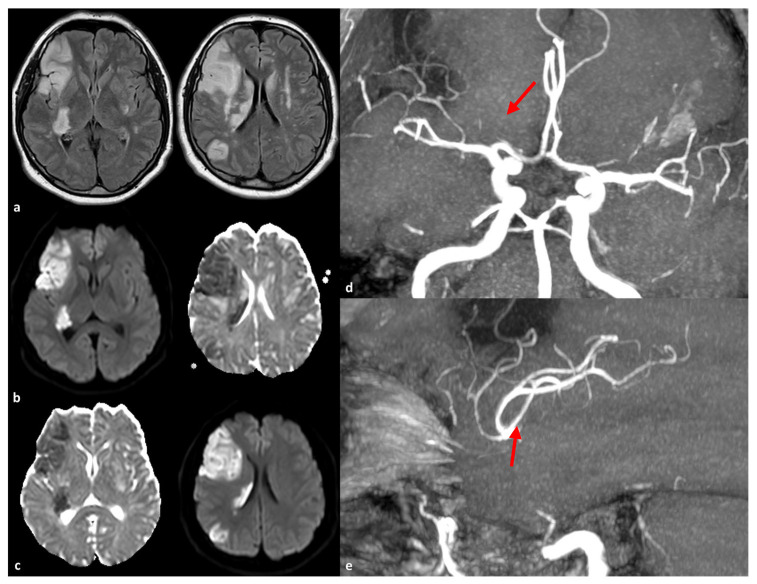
Brain MRI of a patient with a heterozygous *NOTCH3* new frame-shift mutation frame-shift p.Cys1344Leufs (Ex24). The diagnosis was performed after admission to the Stroke Unit due to acute stroke in the right MCA territory (panel (a): axial FLAIR; panel (b): DWI; panel (c): ADC map) with multiple DWI-positive territorial lesions. The contralateral hemisphere showed symmetrical WMHs in the deep subcortical white matter (head of the caudate nucleus, external capsule, and corona radiata). On MR angiography (time of flight), reconstructed using maximum intensity projections/multiplanar reconstruction (MIP/MPR) protocol, a residual thinning of the frontal-opercular right MCA branch is evident (panels (d) and (e), red arrow).
